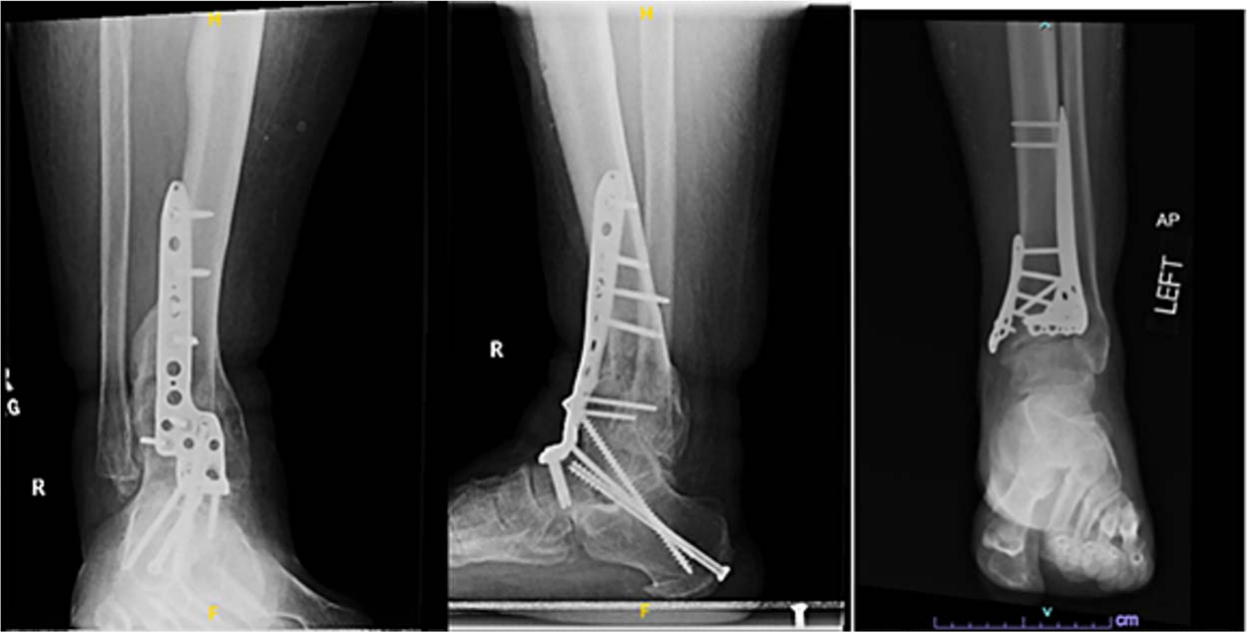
Radiographs of patient 1 obtained 3 years after the index procedure, including AP view of the left ankle and lateral and oblique views of the right ankle following subtalar fusion.
